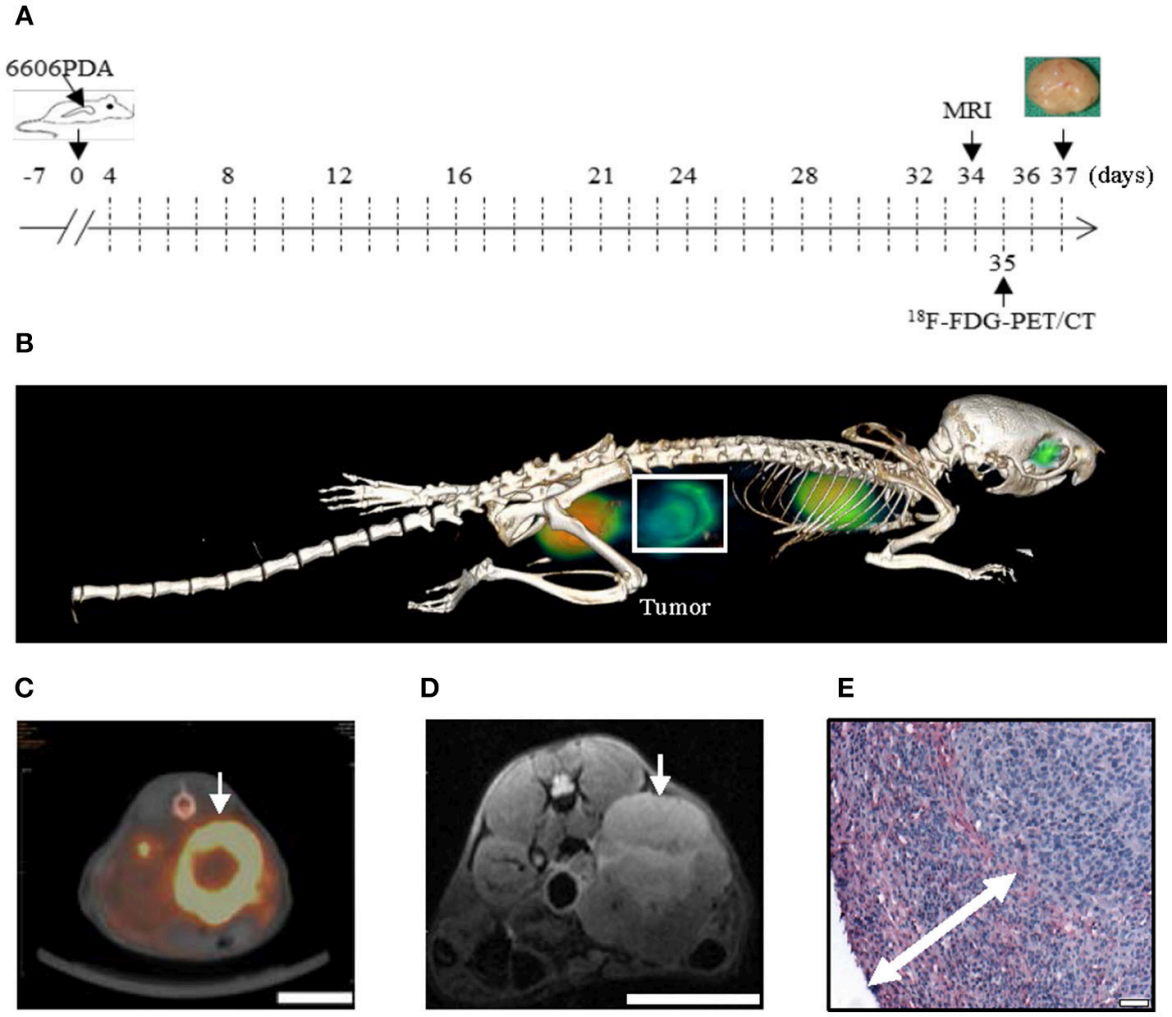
The 6606PDA syngeneic orthotopic pancreatic cancer model replicates features of human pancreatic cancer. 6606PDA cells were injected into the pancreas on day 0 and the chemotherapy started on day 4 (A). The tumor (white box or arrow) could be identified by ^18^F-FDG-PET/CT on day 35 (B,C) and 7 T MRI on day 34 (D). Tumors are surrounded by abundant collagen I deposition as indicated by double arrow (E). Bar = 1 cm in (C,D). Bar = 50 μm in (E).
